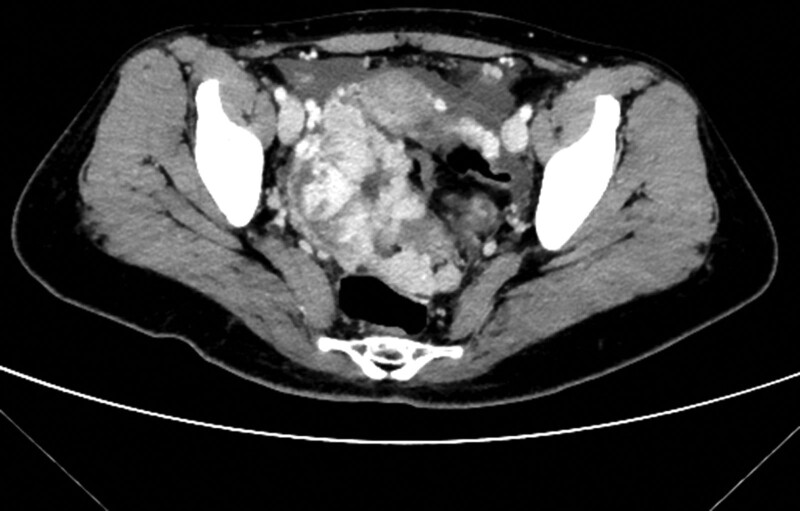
Pelvic CT showing implant metastases. CT = computed tomography.
